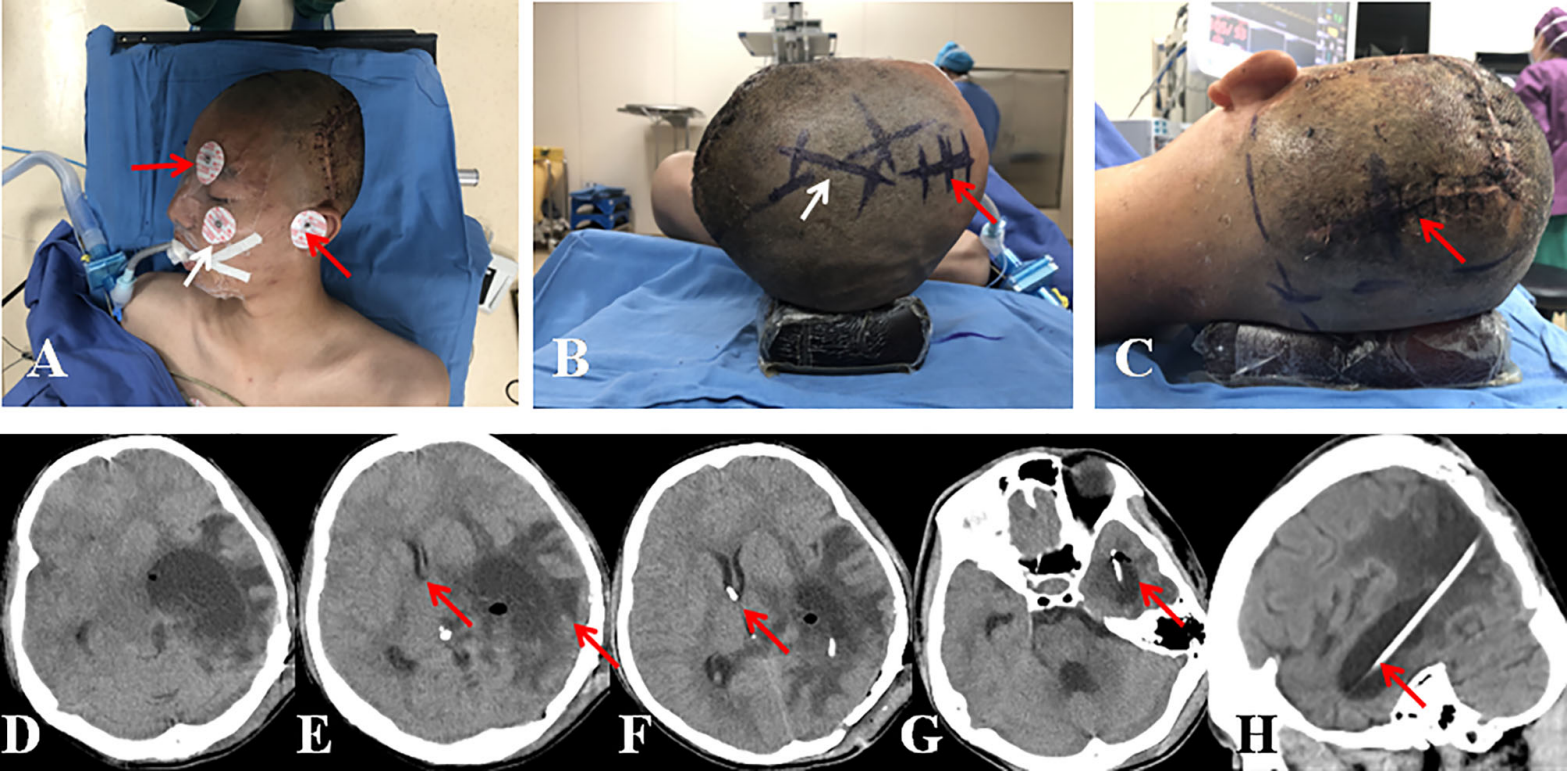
(A) Patient position in superior view (red arrow, landmarks of medial canthus and tragus; white arrow, landmark of zygomatic process). (B) Patient position in rostral view (red arrow, skin incision for frontal burr hole; white arrow, skin incision for subcutaneous connection of the temporal and frontal catheters). (C) Patient position in left view (red arrow, skin incision for occipito-parietal burr hole). (D) CT scan showed severe dilation of the left temporal horn with compression of the brainstem. (E) CT scan showed prominent midline shift, PVBE, and displaced frontal horn (red arrows). (F, G) CT scan showed the suggested localization of catheter tips in the frontal and temporal horns (red arrows). (H): CT scan showed the trajectory of temporal catheter (red arrow).
